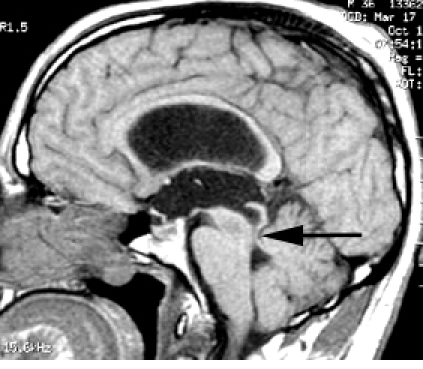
MR image demonstrates an aqueductal stenosis, like an intracranial hypertension determined by disorders of cerebro–spinal fluid circulation
